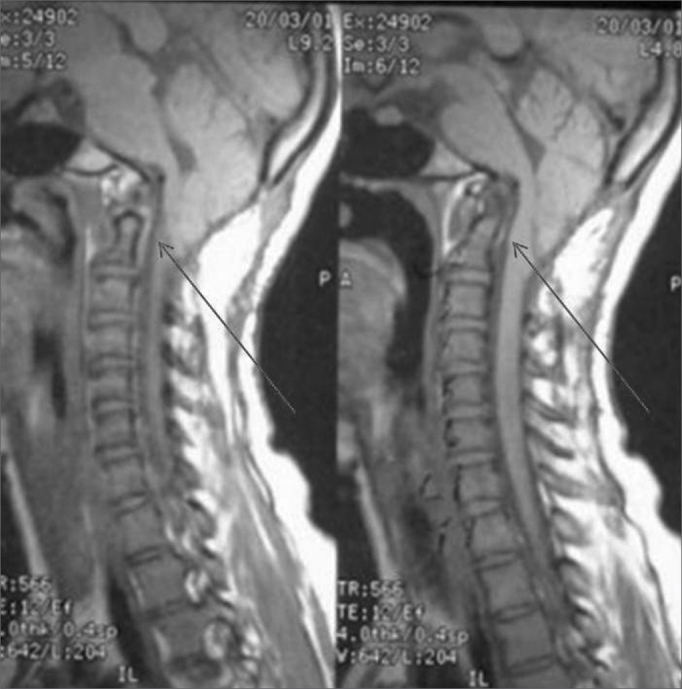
Magnetic resonance image showing brainstem compression by the odontoid process.
